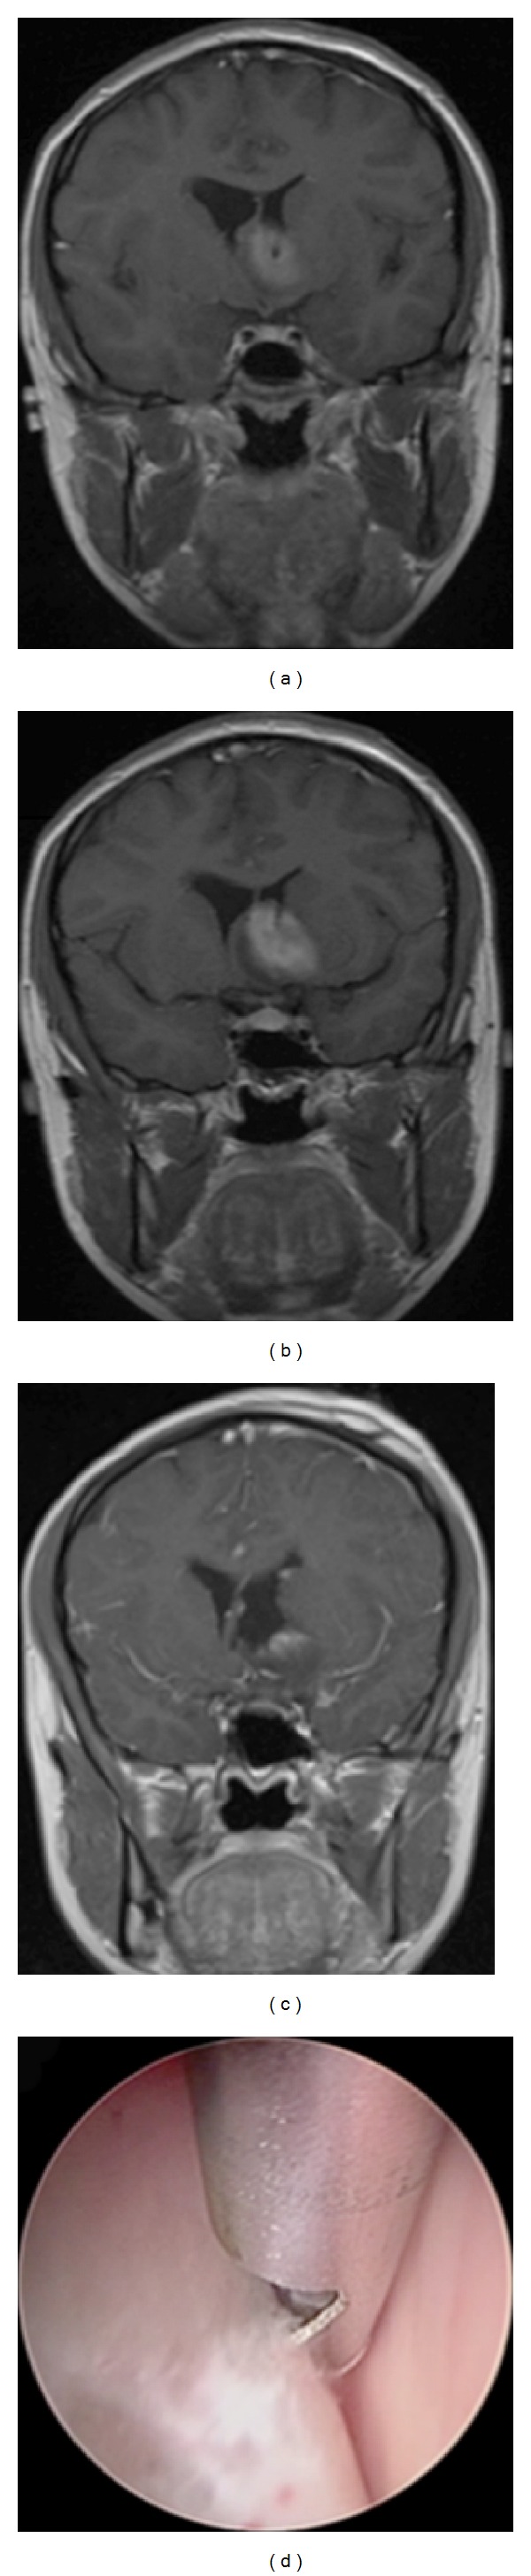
Patient 14, pilocytic astrocytoma. ((a) and (b)) Preoperative coronal T1-weighted contrast-enhanced magnetic resonance imaging showing enhancing lesion and obstructive hydrocephalus. (c) Decrease in ventricular size with interval debulking of lesion. (d) Intraoperative endoscopic view of the tissue aspirator resecting tumor.
